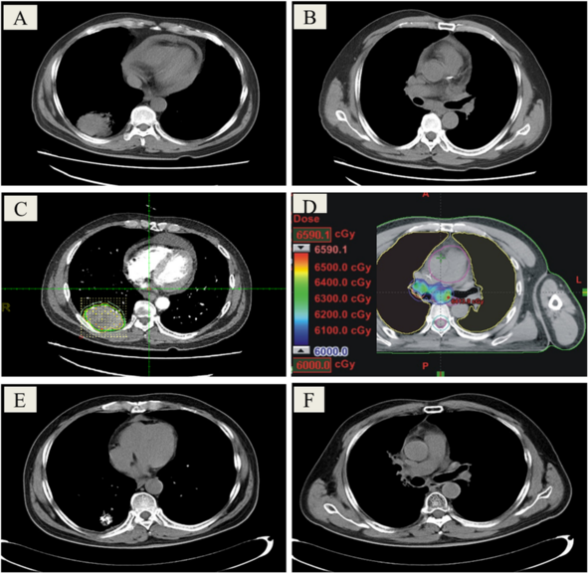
CT images of moderately differentiated squamous cell carcinoma (4 × 5 × 5 cm^3^) in the lower right lung (a) with metastasis in the lymph nodes of the mediastinum (b) in a 64-year old man (No. 18). The patient received iodine-125 seed implantation for lung tumor (c) and external beam radiotherapy for lymph node metastasis (d). Complete disappearance of the lung tumor (e) and lymph node metastasis (f) was observed 1 month after treatment
